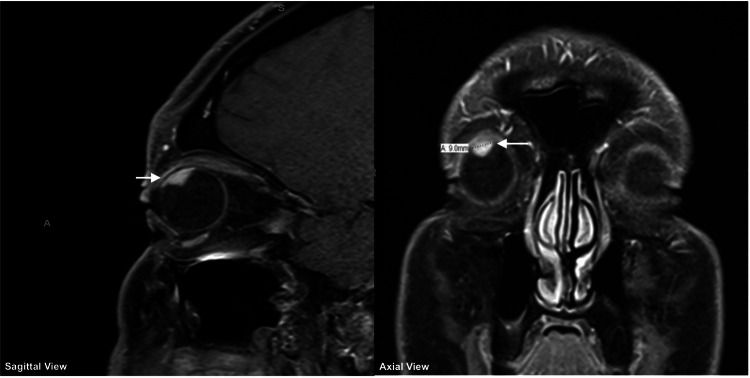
MRI of patient with right 9 mm (COMS-medium) uveal melanoma (sagittal, axial views) COMS: Collaborative Ocular Melanoma Study
